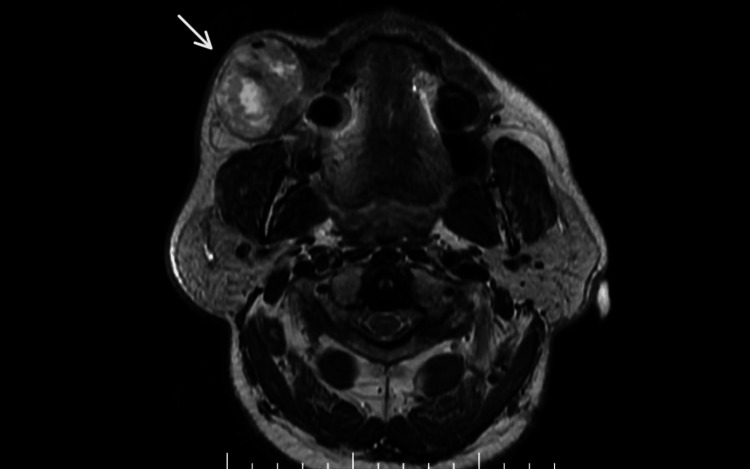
Magnetic resonance imaging showing a well-circumscribed, predominantly solid, heterointense lesion in the subcutaneous plane of right cheek The lesion was located along the course of Stensen’s duct, causing elevation and compression of the duct with proximal sialectasis. The white arrow indicates the location of the lesion.
